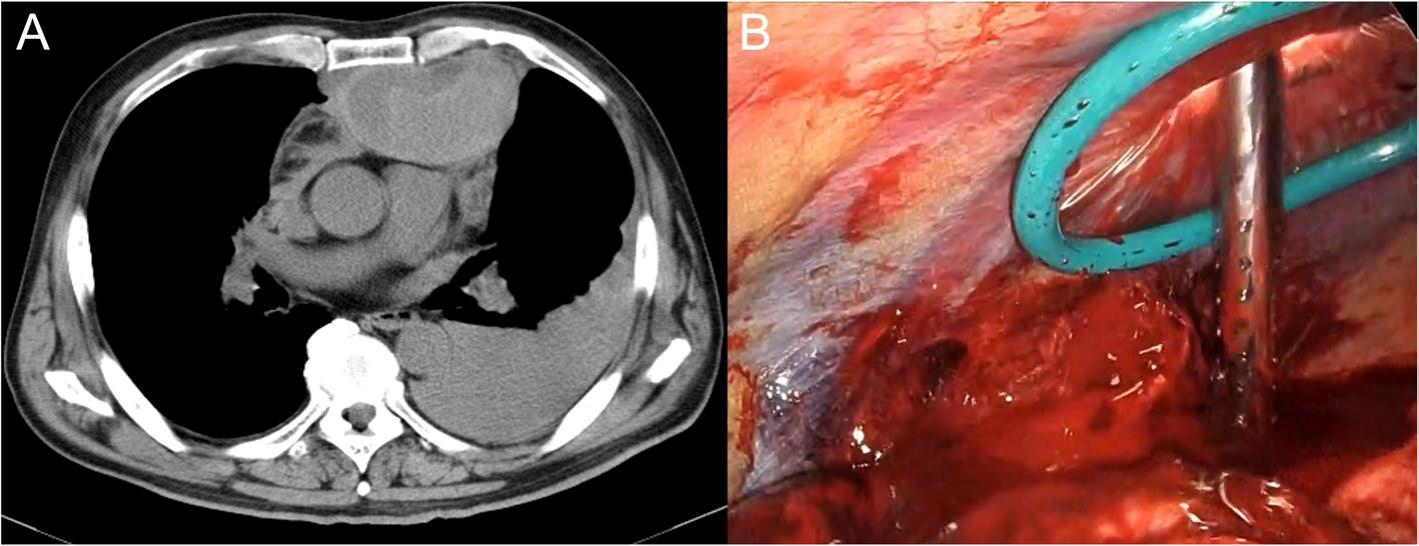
Preoperative and intraoperative findings. A Preoperative computed tomography shows a hematoma in the anterior mediastinum and fluid accumulation in the left thoracic cavity. B Bleeding from a right internal thoracic vein branch was suspected; however, the bleeding point was unclear
